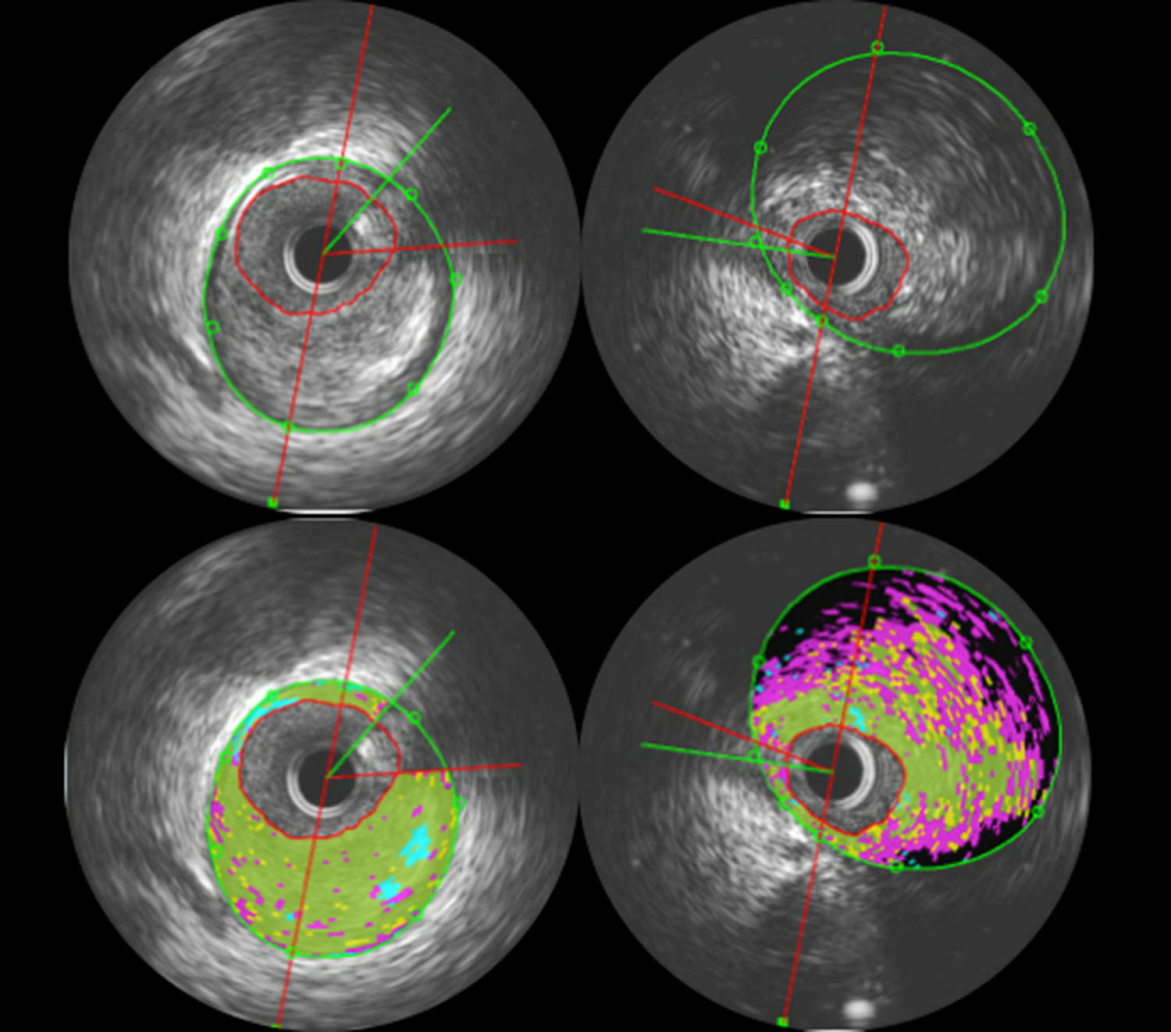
The technique of intravascular ultrasound (IVUS) and iMap classifies the plaque tissue into four color-coded components: green (fibrous), yellow (lipid), pink (necrotic), and blue (calcified). The software used for analysis allows exclusion of the wire shadow from tissue characterization as unknown plaque (*black*) and/or exclusion of the tissue behind calcification
